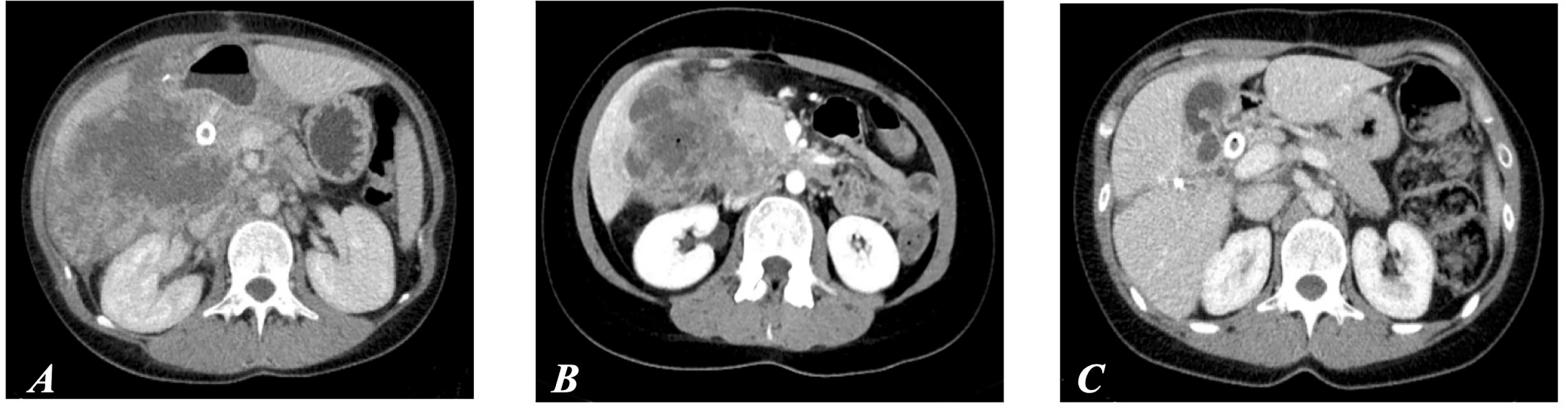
(A) CT scan performed before surgery on primary tumor. (B) CT at day 42, after the first cycle of immunotherapy. (C) CT after six cycles of immunotherapy (first evidence of partial response according to RECIST 1.1 criteria).
